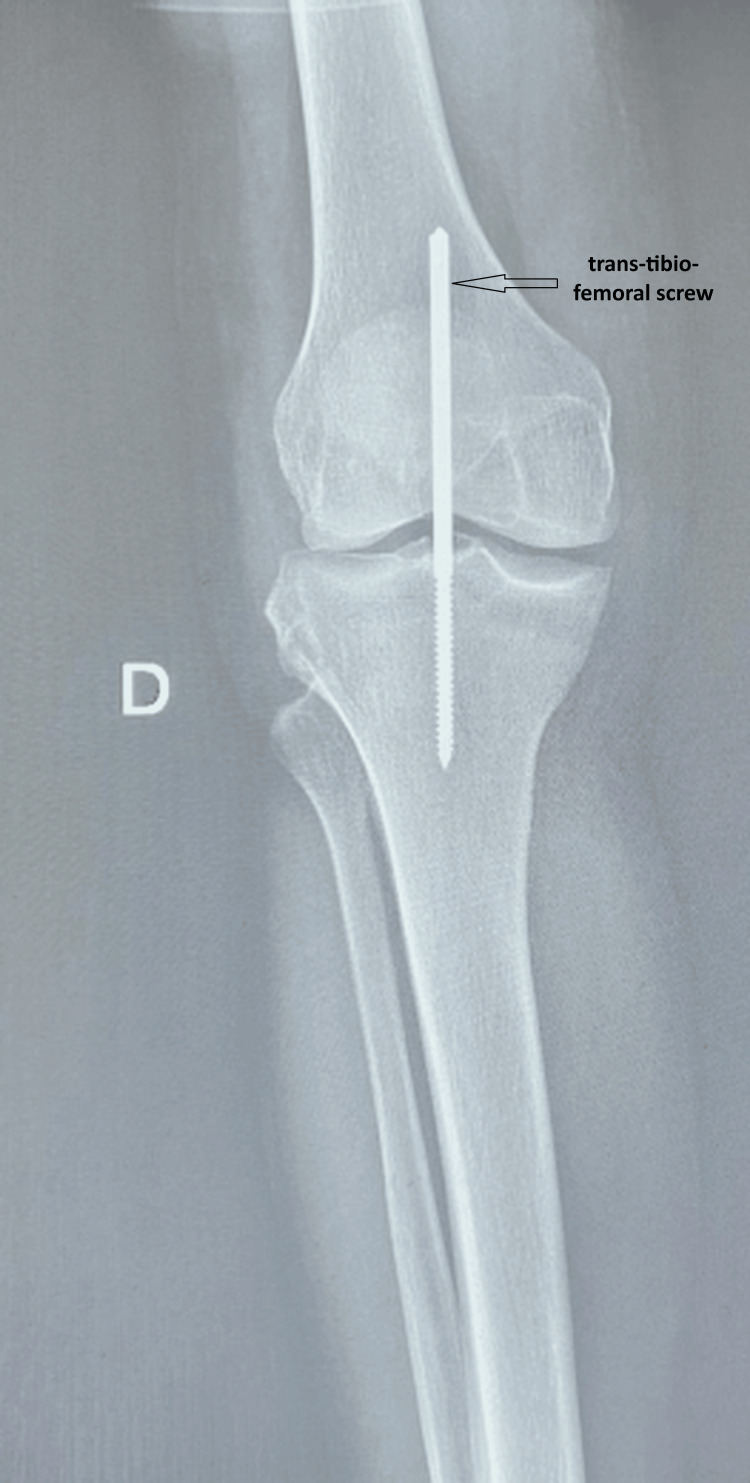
Standard radiograph of the right knee after reduction and stabilization with a trans-tibio-femoral screw
